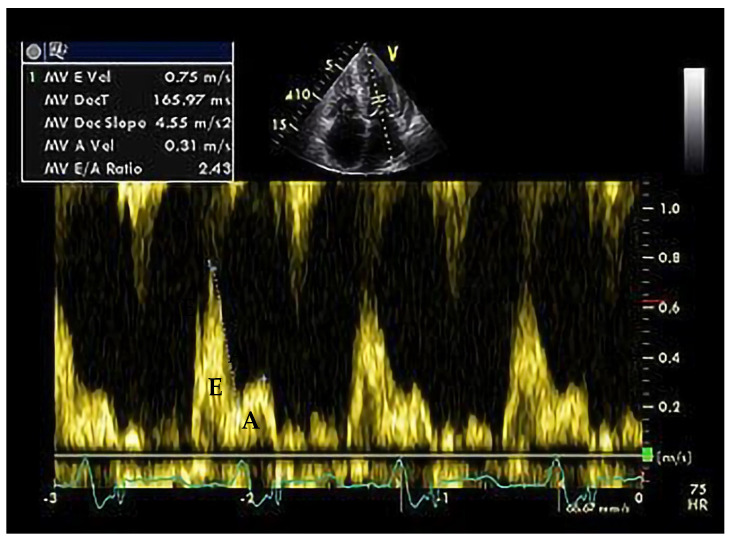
Pulsed Wave Doppler (PWD) at the mitral valve (MV) level, with E/A ratio of 2.4—suggestive for restrictive diastolic dysfunction.
